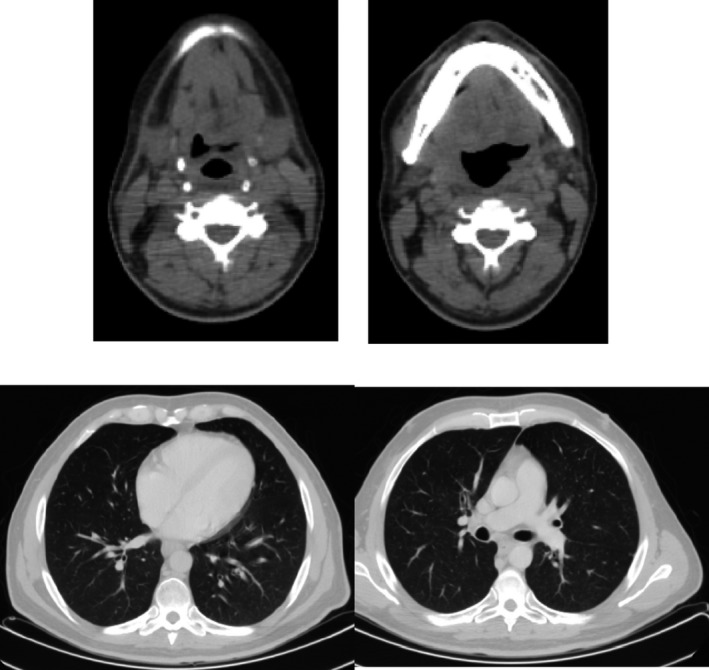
February 2014; second restaging after chemoradiation (CT scan).
